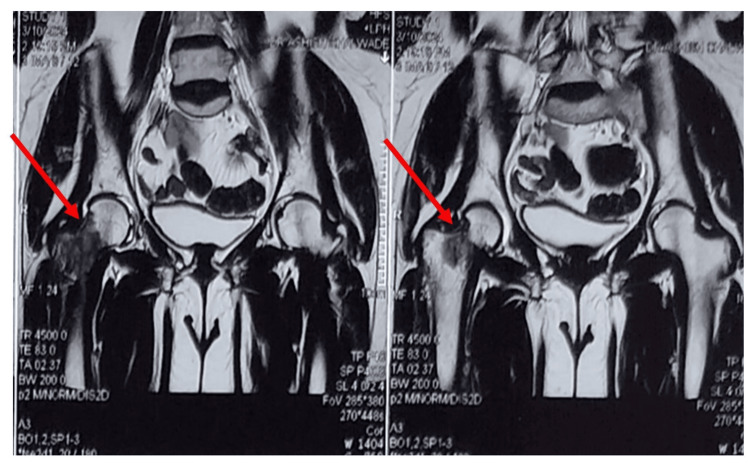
Coronal MRI pelvis with both hips cuts showing proximal femoral metastasis The red arrow marks show a hypointense lesion in the neck and intertrochanteric area.
